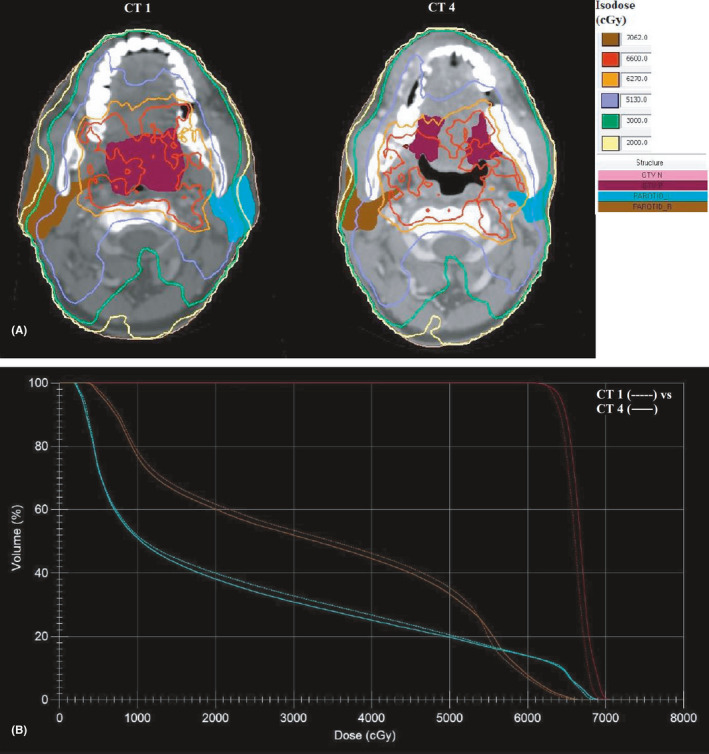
A, Axial CT sections of a T2N0 soft palate carcinoma. Dose distribution of Plan 1 on CT1 (left) and on CT4(right). Patient lost about 6 kgs during treatment. The parotid glands decreased in size during treatment. The 30 Gy (light green) and 20 Gy (light yellow) isodose lines shifted closer to lateral border of the parotid glands in CT4 compared to CT1. B,. DVH comparison between CT1(
